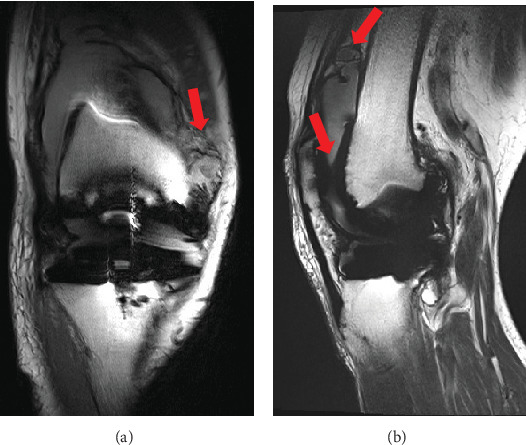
Magnetic resonance imaging in the right knee at 4 years after surgery. (a) Coronal view and (b) sagittal view of the right knee T2 low imaging demonstrating a moderate knee effusion (red arrow) and a low signal–high signal with hemorrhage in the suprapatellar and medial pouch (red arrow).
